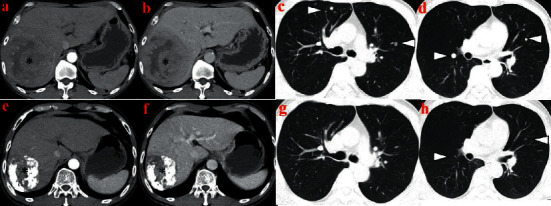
Representative imaging in advanced HCC patients receiving TACE combined with camrelizumab plus apatinib. (a-b) Enhanced abdominal CT showing massive HCC (asterisk). (c-d) Chest CT showing multiple lung metastases (white arrowhead). (e–h) At 3 months after combination therapy, follow-up CT demonstrated a partial response, with the tumor shrinking significantly in the liver (asterisk) and lung (white arrowhead).
